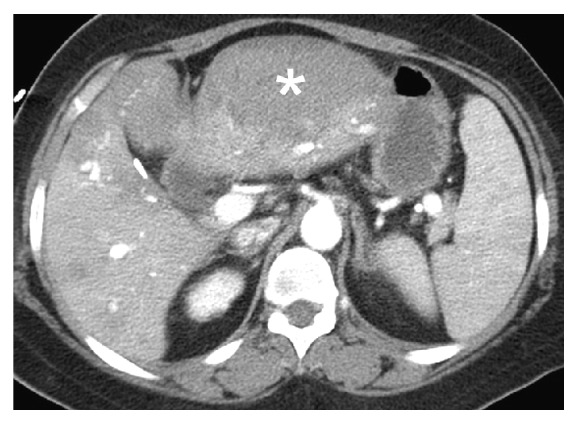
The computed tomographic angiography of abdomen of first time revealed subcapsular hematoma (asterisk) with contrast extravasation at left lateral segment of liver.
